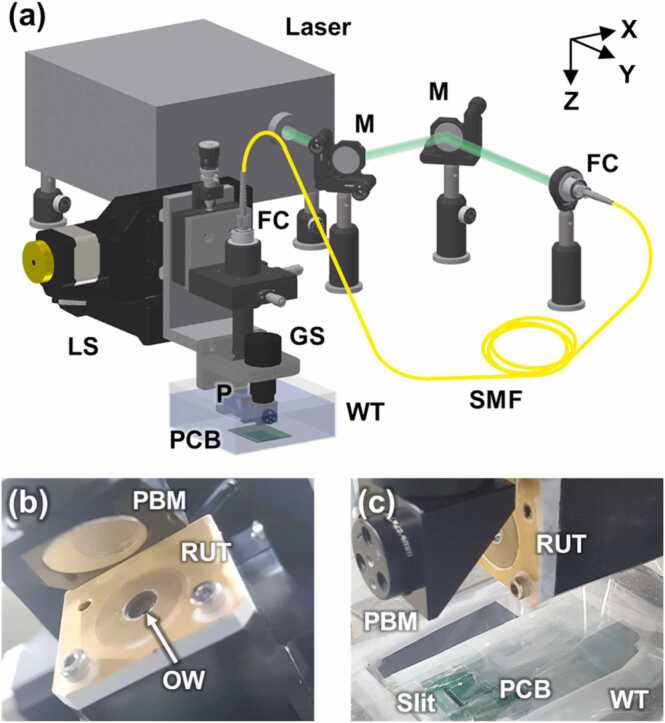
(a) Graphical representation of the combined PAM-PPG system. (b–c) Photographs of (b) the flat ring-shaped ultrasound (US) transducer, parabolic mirror, and PPG PCB wrapped in plastic wrap for waterproofing and attached on water tank. M, mirror; FC, fiber coupler/collimator; SMF, single-mode optical fiber; P, prism; GS, galvanometer scanner; WT, water tank; LS, linear stage; RUT, ring-shaped US transducer; OW, optical window; PBM, parabolic mirror; and PCB, printed circuit board.
